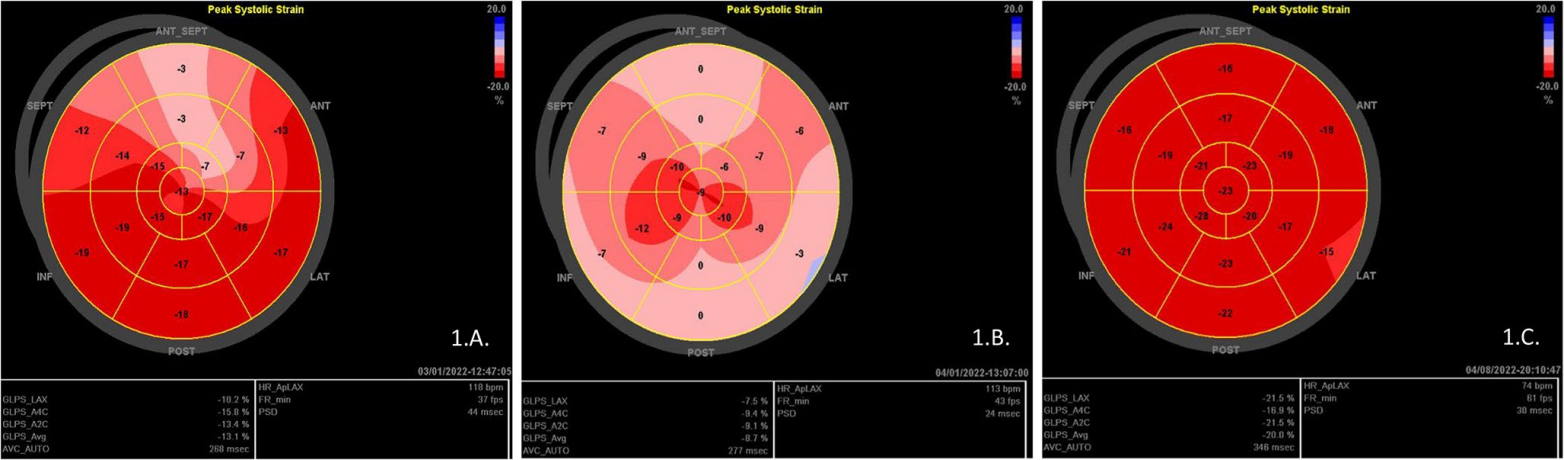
Evolvement of myocardial strain abnormalities detected on a patient diagnosed with MIS-C. At admission, while the patient had normal systolic function parameters assessed by conventional Echocardiography, strain rate was abnormal on the anterior septal segment of the left ventricle (A). Three days after the admission, strain measurements further deteriorated, demonstrating generalized global hypokinesis of the left ventricular wall (B). Seven months later, there was full resolution of the myocardial dysfunction, with normalization of the systolic and diastolic function (C). The bull’s eye of the strain rate was normal (C)
